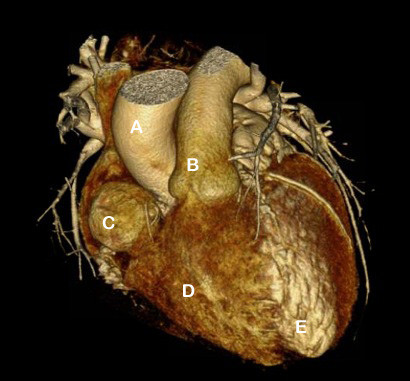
Cardiac CT image exhibiting the giant right coronary artery aneurysm. A- aorta; B - pulmonary artery; C - right coronary artery aneurysm; D - right ventricle; E - left ventricle.
